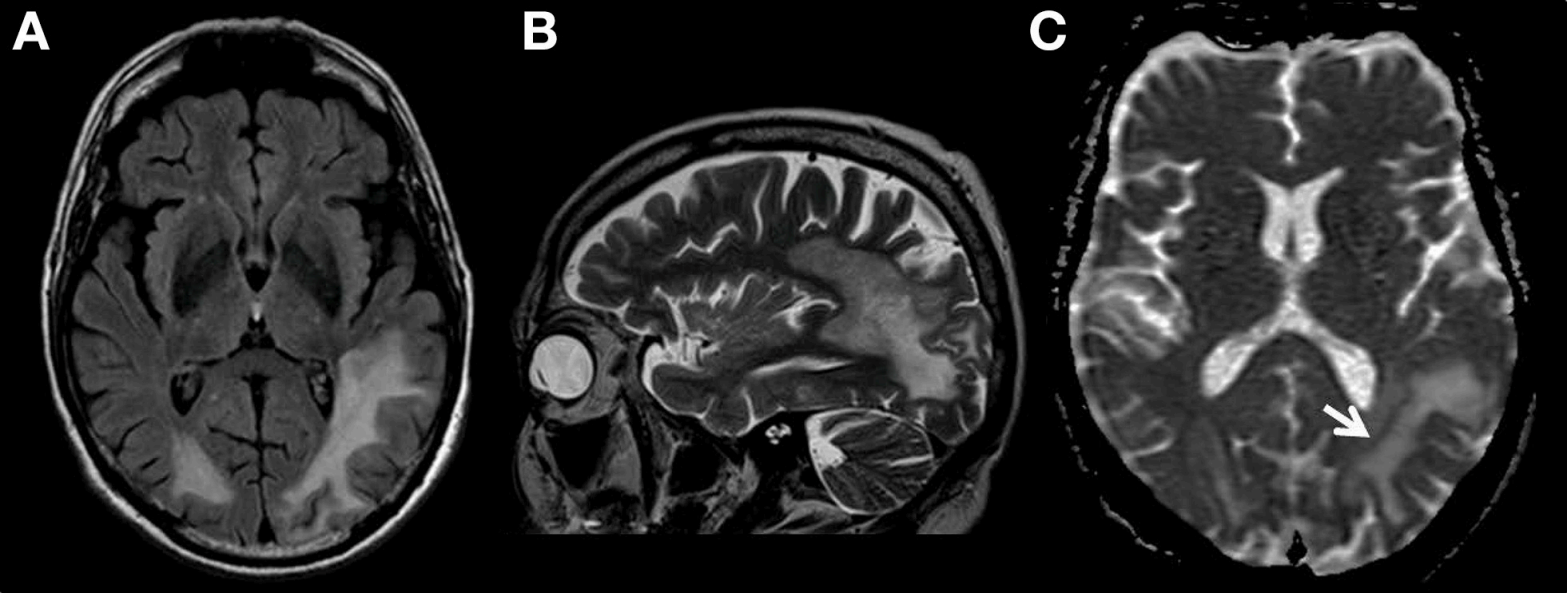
MR examination showing typical findings of PML with white matter FLAIR- (A) and T2 weighted- (B) hyperintensities without mass effect. T2-signal elevations (B) are in part spot-like and resemble the milky-way-appearance typical for PML. The area affected by the JC-virus infection does have a typical rim with reduced ADC values (C, white arrow) indicating the inflammatory border zone with acute demyelination combined with swelling of astrocytes and oligodendrocytes.
